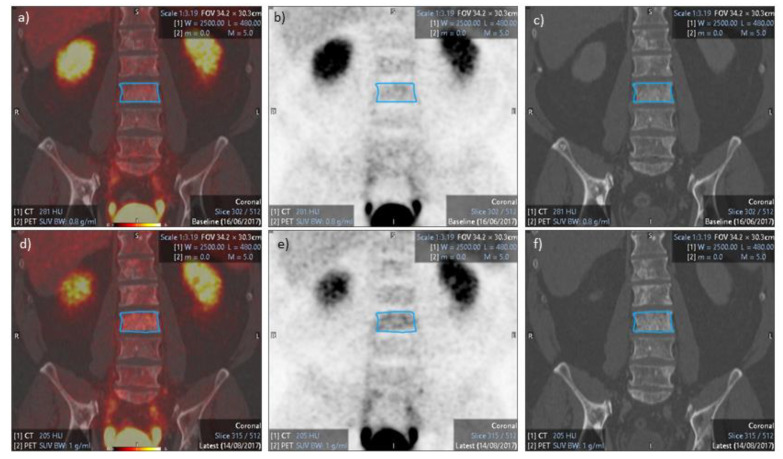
uPAR PET/CT (patient 10) Coronal fused images (left), PET images (centre) and CT images (right). Baseline images (a–c) and follow-up after two cycles of ^223^RaCl_2_ (d–f). Bone metastasis in lumbar spine (L3) is delineated, baseline SUVmax was 2.4 and after two cycles of ^223^RaCl_2_ increased to 3.9. After end of therapy the patient was diagnosed with epidural involvement at L3 and received EBRT to relieve symptoms.
